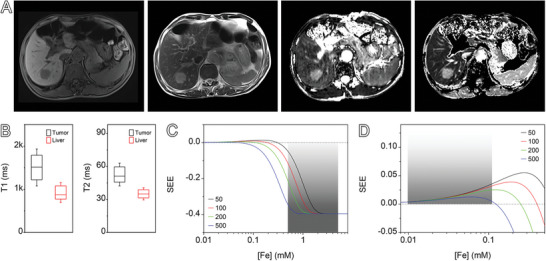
Theoretical SEE prediction for HCC patients. A) Representative T1‐weighted, T2‐weighted, T1 mapping, and T2 mapping images of an HCC patient acquired by MRI scans without contrast agent. B) Relaxation time data for the liver and tumor regions from 15 HCC patients. Data are presented as mean ± SD, *n* = 15. C,D) Theoretical curves depicting SEE as a function of average [Fe] for the liver (C) and tumor (D) at specified enrichment levels, with [Fe]_endosome_ exceeding the average [Fe] in the liver or tumor matrix by 50–500 times.
